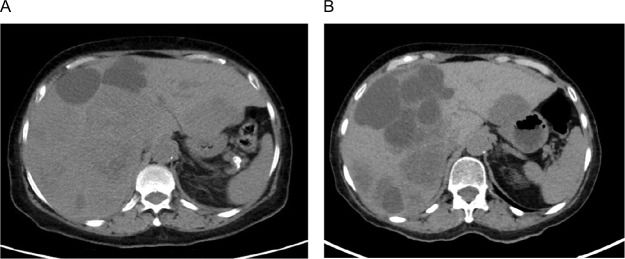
(A) Computed tomography (CT) abdomen and pelvis (pre-treatment) showing hepatomegaly and several hypodense lesions throughout the liver parenchyma consistent with liver metastases. (B) CT abdomen and pelvis (post-treatment) showing enlargement of existing lesions and new hepatic metastases.
